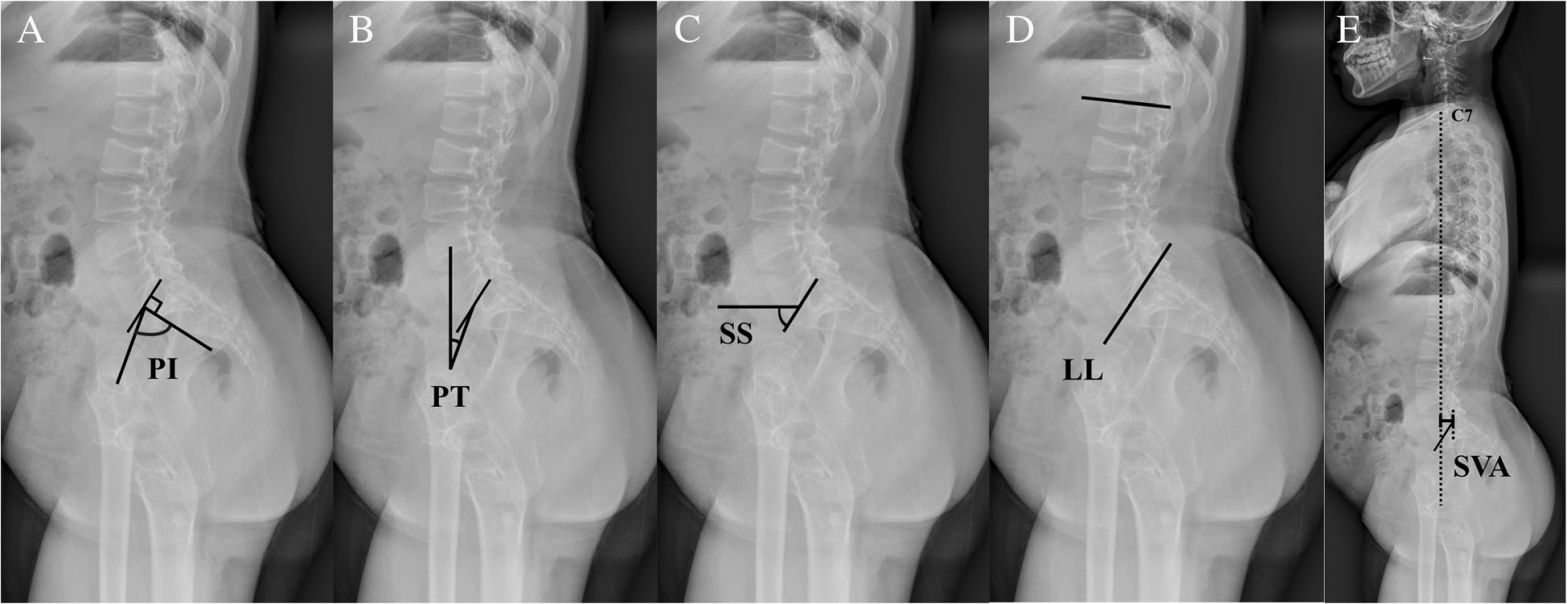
Spinopelvic parameters measured on standing radiographs. a Pelvic incidence (PI) was defined as the angle between a perpendicular line to the sacral endplate and a line joining the middle of the sacral endplate and the hip axis. b Pelvic tilt (PT) was defined as the angle between the line joining the middle of the sacral endplate and the hip axis and a vertical line. c Sacral slope (SS) was defined as the angle between the sacral superior endplate and the horizontal plane. d Lumbar lordosis (LL) was measured between the upper endplate of L1 and the upper endplate of S1 by using the Cobb method. e Sagittal vertical axis (SVA) was defined as the horizontal distance between a plumb line dropped from the center of C7 to the posterior-superior corner of S1
